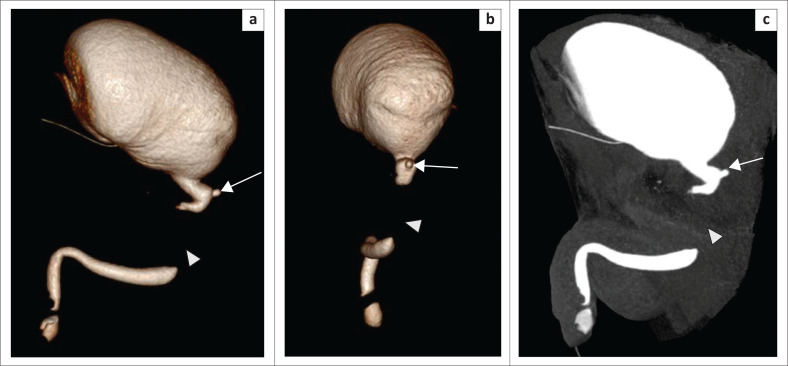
(a, b) Volume rendered technique images (multidetector computed tomographic urethrography) showing a posterior urethral distraction defect (arrowhead) and a urethral diverticulum (arrow) in different planes. (c) Maximum intensity projection image of the same patient showing the posterior urethral distraction defect (arrowhead) and urethral diverticulum (arrow) clearly.
